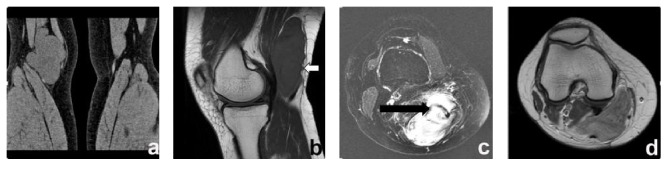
A 47-year-old female with well-differentiated adult fibrosarcoma*. (a) A coronal CT scan exhibited a lobulated iso-attenuating soft tissue mass in the right popliteal fossa. (b) Sagittal T1WI showed the lesion with a nodular lump close to the deep fascia (white arrow). (c) Axial fat suppressed FSE T2WI showed band-like areas of low signal suggesting tumor fibrous septa (black arrow). (d) An axial SE T1W contrast-enhanced scan exhibited heterogeneous peripheral enhancement.
